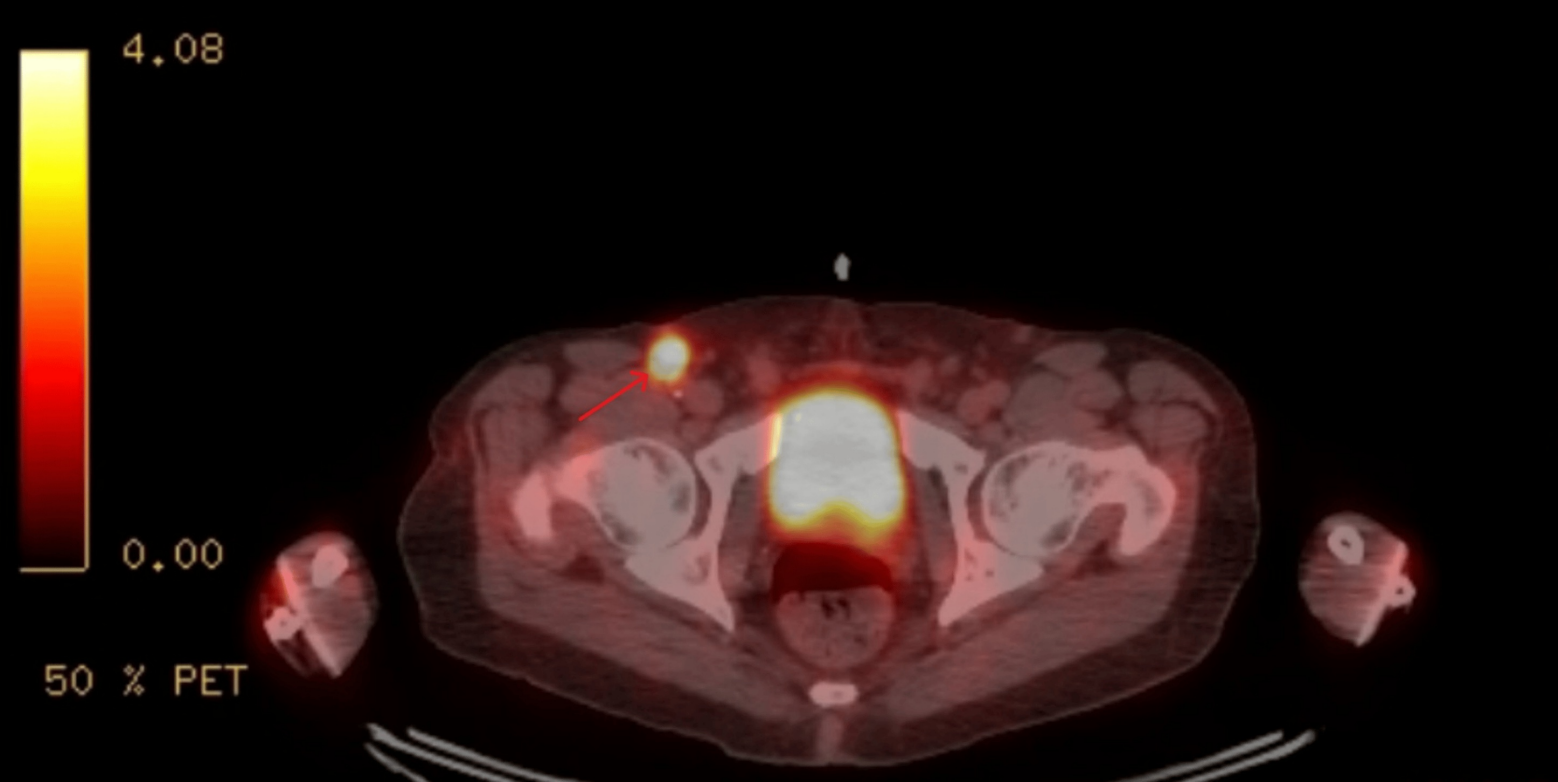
PET-CT showing an intensely FDG-avid 1 cm right inguinal lymph node
